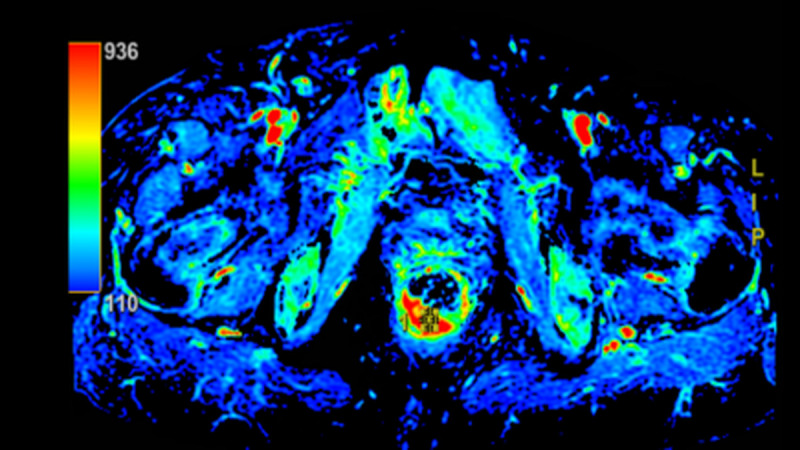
MRI imaging characteristics. Distal rectal lesion (target sign on T2WI, high signal on DWI).
